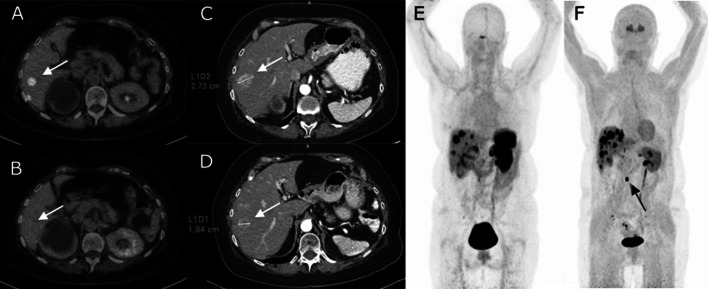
Imaging of a 70-year-old female patient (#2) with a metastasized primary colon NEN (G2). Fourteen tumours were found, of which 11 only by [18F]DOPA PET/CT and three by both PET tracers. PET/CT shows tumour uptake of [18F]DOPA in the liver (A), while [68 Ga]DOTA-TOC shows none (B). The same tumour had a longest axis diameter of 27 mm on the pre-PRRT CT scan (C), reduced to 18 mm in the post-PRRT CT scan (D). These images display significant therapy effect of PRRT in a tumour only found by [18F]DOPA. Maximum intensity projection (MIP) images show higher incidence of focal uptake of [18F]DOPA (F) in the liver compared to [68 Ga]DOTA-TOC (E). A positive para-aortal lymph node was found only by [18F]DOPA (F, black arrow)
